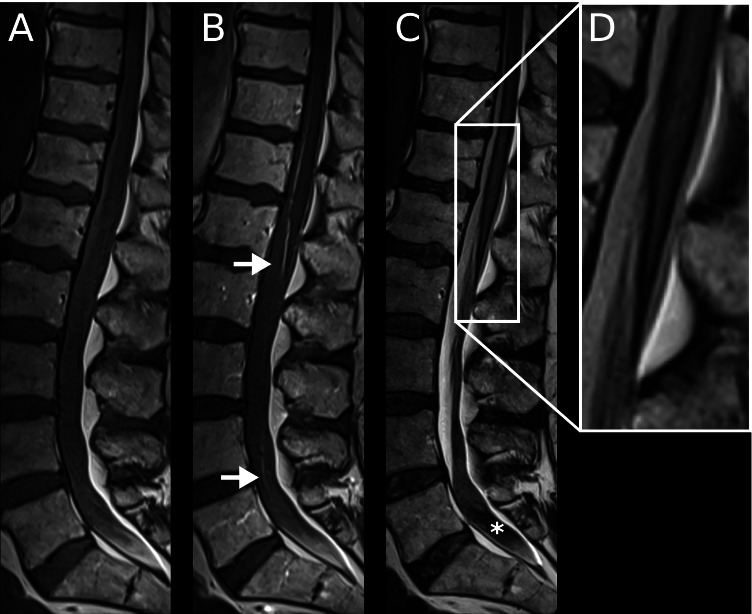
Sagittal pre- A and post-contrast B T1-weighted MR images of the spinal cord, acquired May 2020, show abnormal linear enhancement along the cord surface and diffuse enhancement of the nerve roots of the cauda equina (B, →). Sagittal T2-weighted images C and D show intramedullary cord hyperintense edema and hypointense hemosiderin deposition along the spinal cord surface. The thecal sac shows hypointense blood-fluid levels C,*
